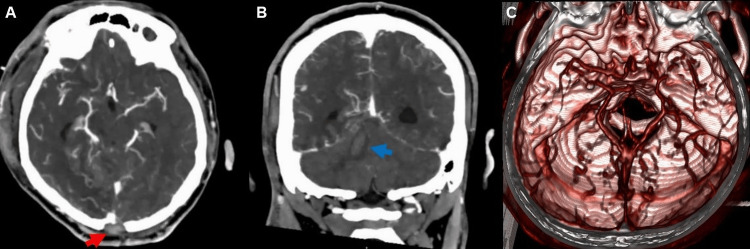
(A) Postoperative axial contrast CT shows changes in the occipital bone (red arrow), and absence of hemorrhage is noted. (B) Edema is found along the trajectory of the knife (blue arrow). (C) 3D vascular reconstruction showing no evidence of injury.
